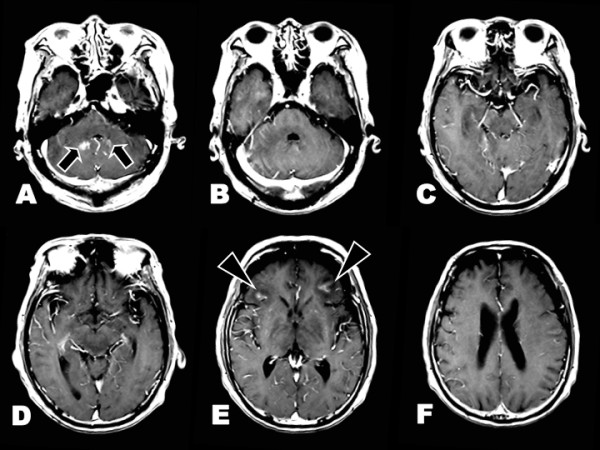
Gadolinium-enhanced T1-weighted imaging. A, E. Gadolinium enhancement are found in the cerebellum (arrows) and bilateral frontal subcortex (arrowheads). B-D, F. There is no enhancement in the brainstem and basal ganglia.
